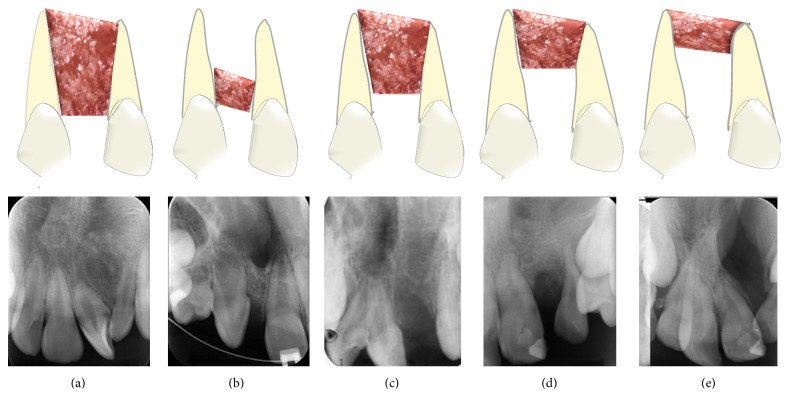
Periapical radiographic images of autogenous bone grafts according to the Chelsea classification. (a) Type A: the presence of bone tissue at the cementoenamel junction of the teeth adjacent to the cleft and at least 75% of both roots covered by bone. (b) Type B: the presence of bone tissue at the cementoenamel junction of the teeth adjacent to the cleft and at least 25% of both roots covered by bone. (c) Type C: the presence of bone tissue surrounding at least 75% of the roots in the cleft area with an apical direction. (d) Type D: the presence of bone tissue surrounding at least 50% of both roots in the cleft area, with an apical to coronal direction. (e) Type F: the presence of 25% or less of bone tissue in both roots in the apical direction.
